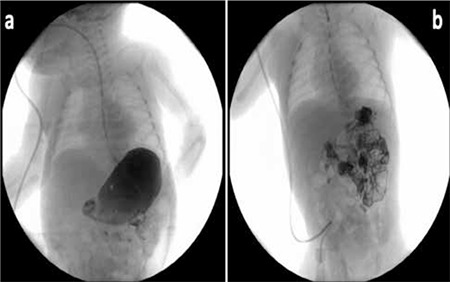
Upper gastrointestinal system radiography (UGIR) prior to L-thyroxine treatment: Upper GI series performed by using diluted barium via feeding catheter demonstrates a dilated and distended stomach at the 15th minute of the examination. There is slight opacification of the duodenum, and the emptying time of the stomach is markedly delayed. b) UGIR subsequent to L-thyroxine treatment: Follow-up GI series after medical treatment demonstrates that the emptying time of the stomach has improved. The stomach has almost totally emptied at the 15th minute of the examination with opacification of the duodenum and proximal jejunum
